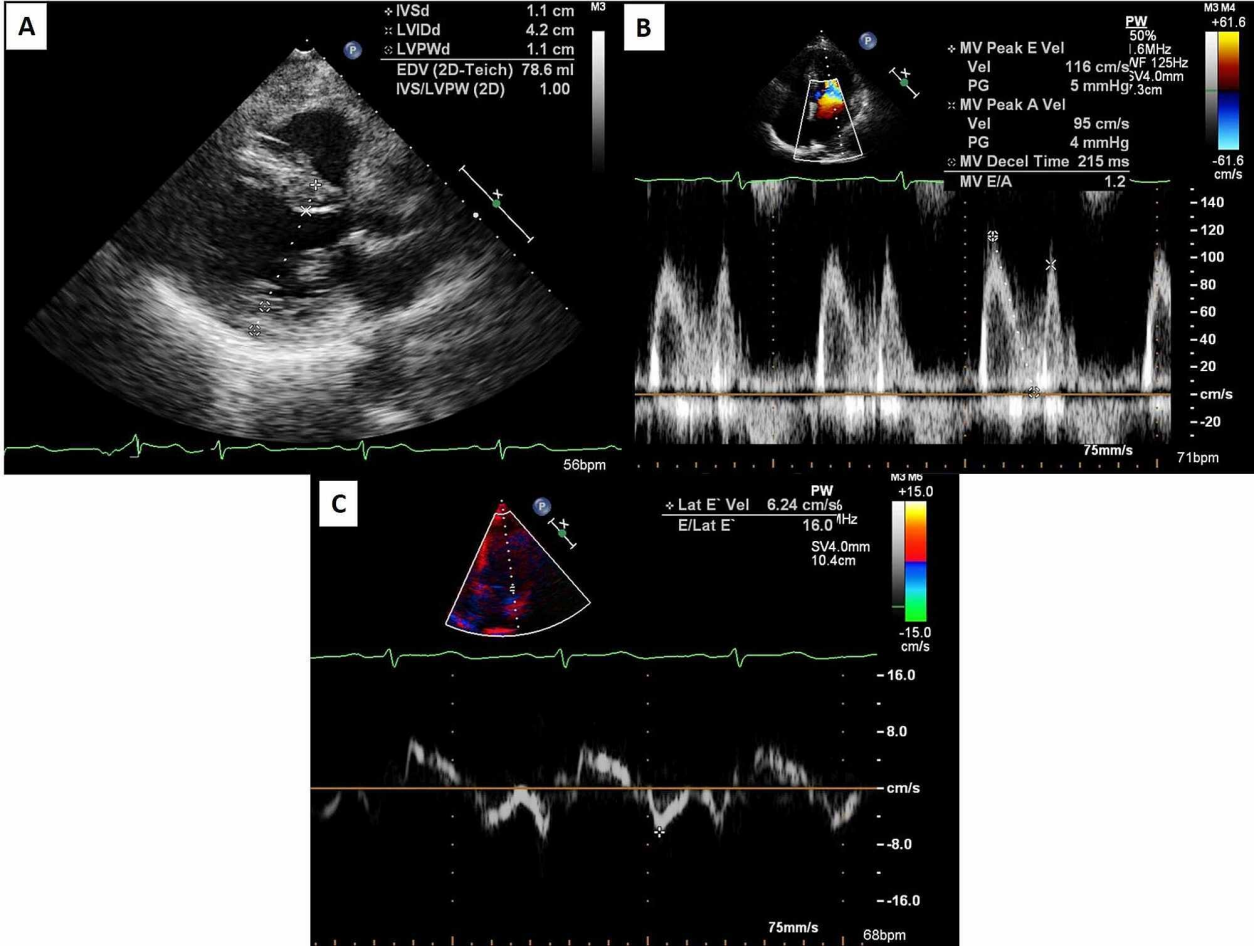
Echocardiogram. A:  Left ventricular wall thickness is demonstrated here using the parasternal long-axis view of the heart at end diastole. Septal thickness and posterior wall thickness are both measured at 1.1 cm which is higher than the normal range of 0.6-0.9 cm for both in females. B: Filling pressures are ascertained using doppler data gathered across the mitral valve in the apical view. The E and the A refer to two distinct waves created by the passive and active filling of the left ventricle respectively. Our patient's E/A ratio is 1.2 which is in the normal range of 0.75 and 1.5 making this a pseudonormal filling pattern. C: Measuring tissue doppler can provide the E/E' ratio which can help distinguish between normal and pseudonormal filling patterns. Our patient's E/E' shown here is 16 which is highly suggestive of elevated filling pressures. For reference, normal E/E' ratios are less than 8.
